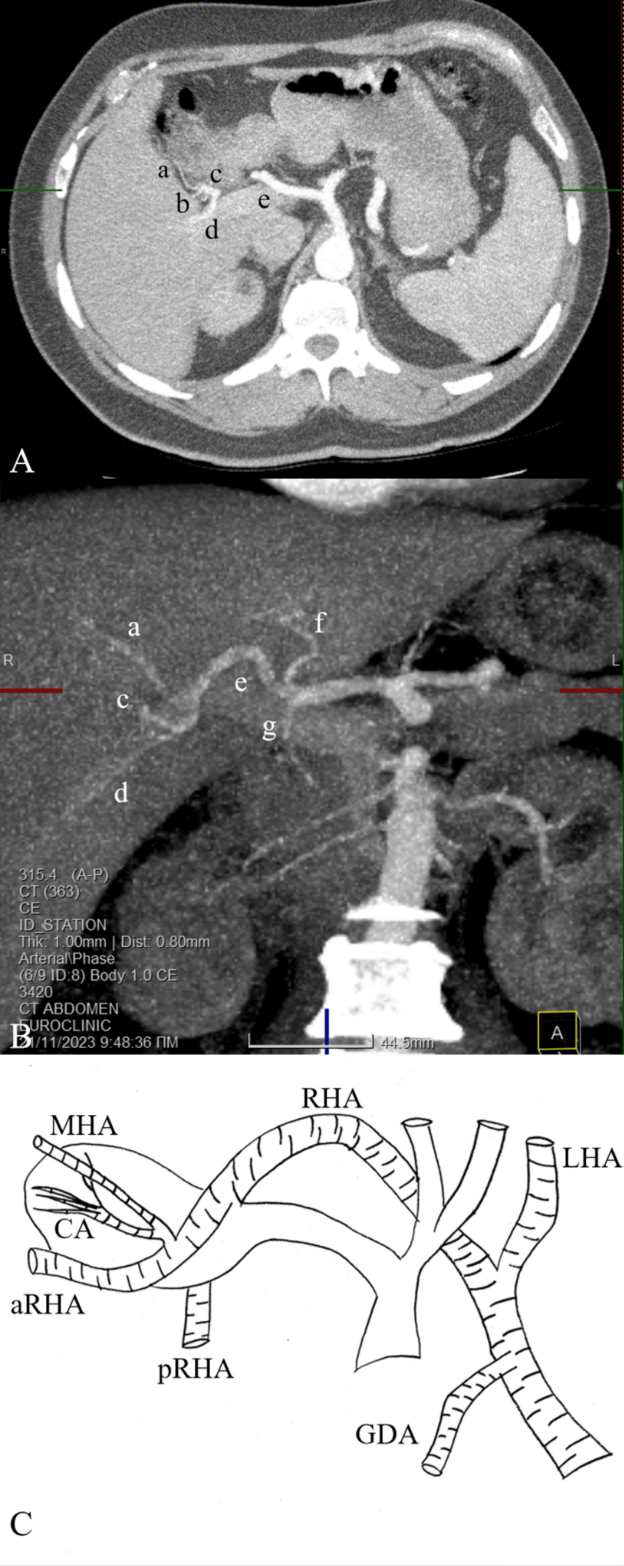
CT (A) coronal plane and (B) traverse plane, and (C) graphical image (a) Middle hepatic artery (ΜΗΑ), (b) cystic artery (CA) (clipped), (c) anterior branch of the right hepatic artery (aRHA), (d) posterior branch of the RHA (pRHA), (e) RHA, (f) left hepatic artery (LHA), (g) gastroduodenal artery (GDA) Image credit: Miltiadis Perdikakis
